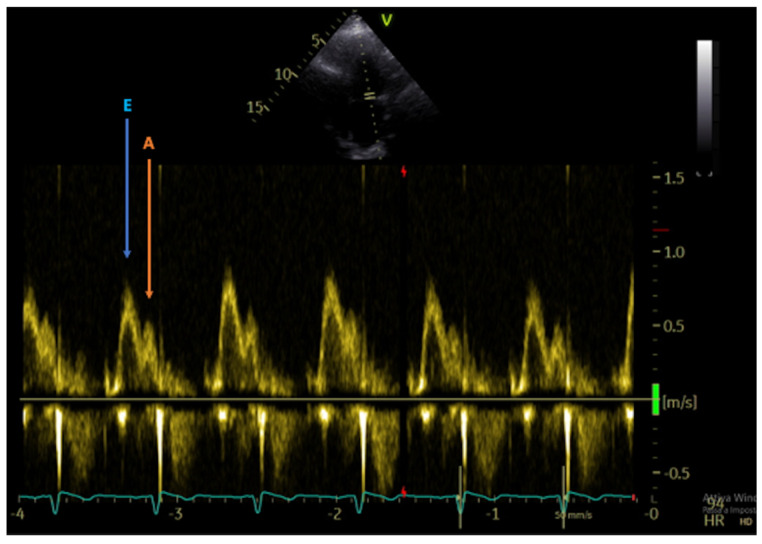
Doppler image showing the overlap of E wave (blue arrow) and A wave (orange arrow) due to the elevated heart rate (HR 94 bpm).
